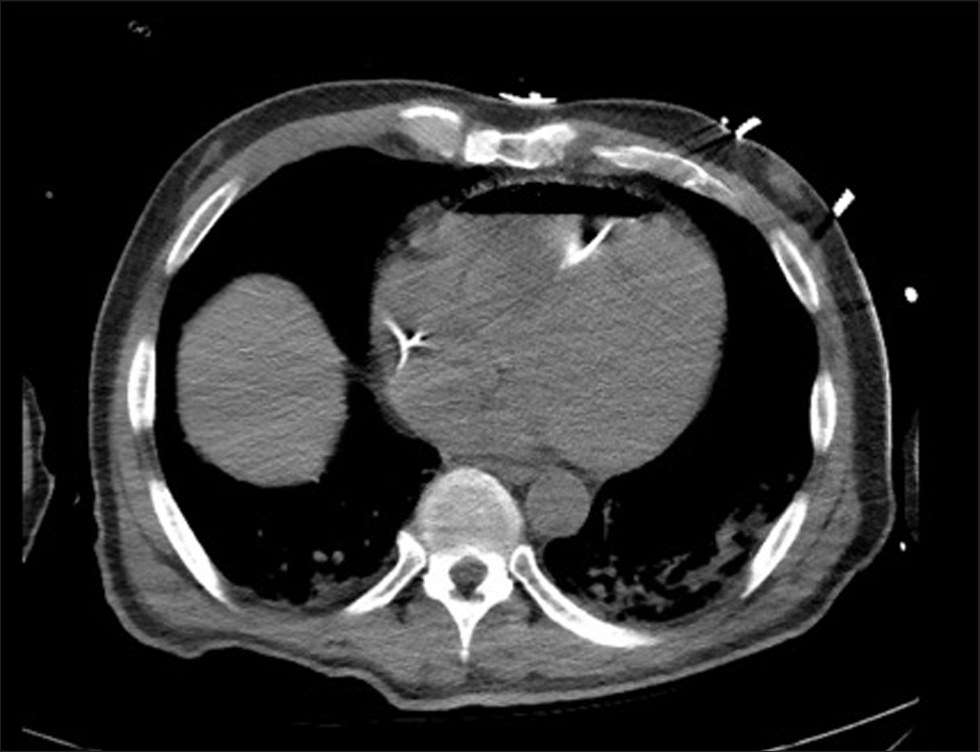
Computed tomography chest axial view showing presence of air in the right ventricle.
